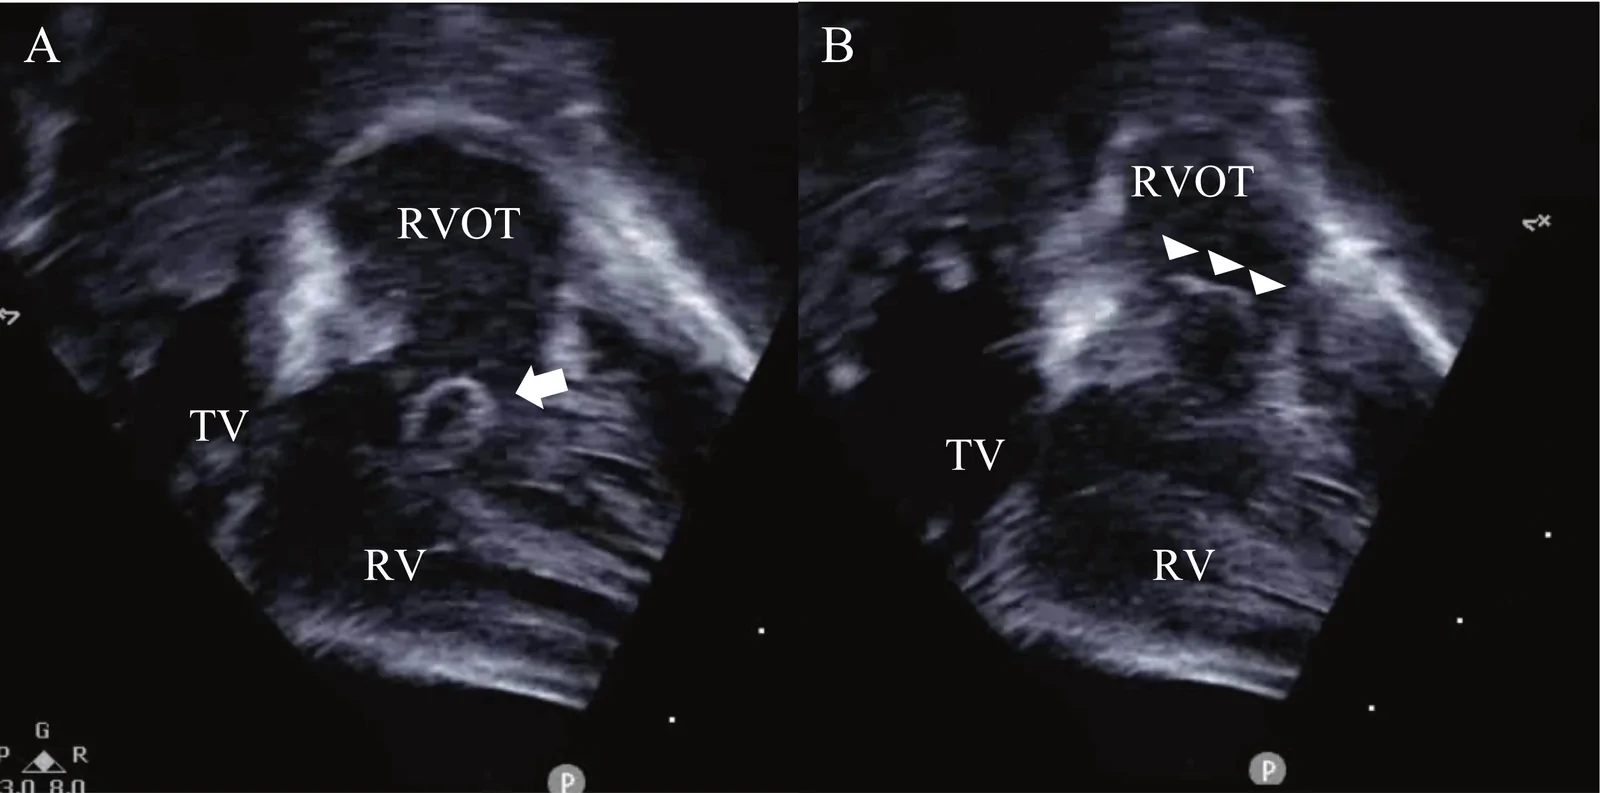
(A) Echocardiographic image of the right ventricle (RV) in the subxiphoid view, showing accessory tricuspid valve (TV) leaflet contiguous with the septal leaflet in diastole (arrow). (B) Accessory TV leaflet prolapsing into the right ventricular outflow tract (RVOT) in systole (arrowhead).
